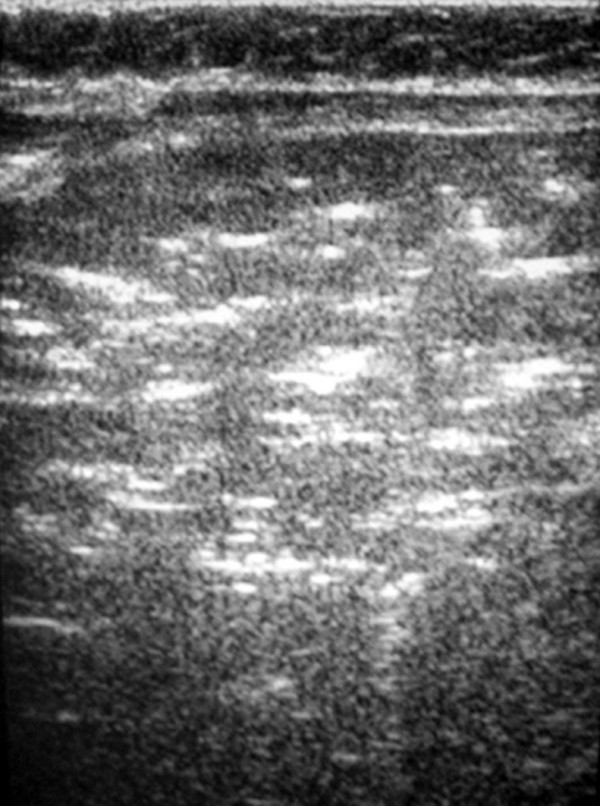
Ultrasonography showing free air bubbles in the hepatic blood vessels.
